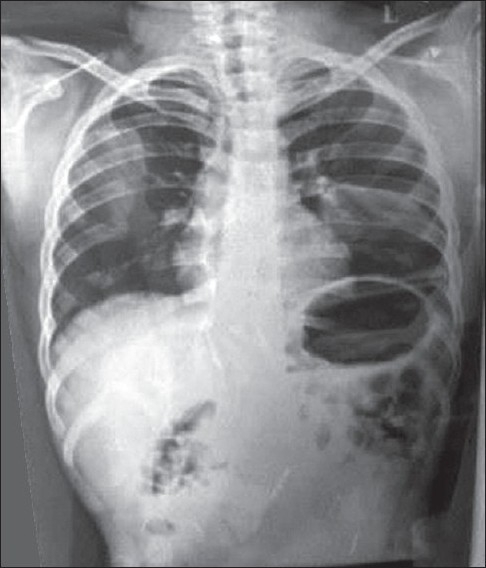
Chest X-ray revealing bilateral cervical ribs
